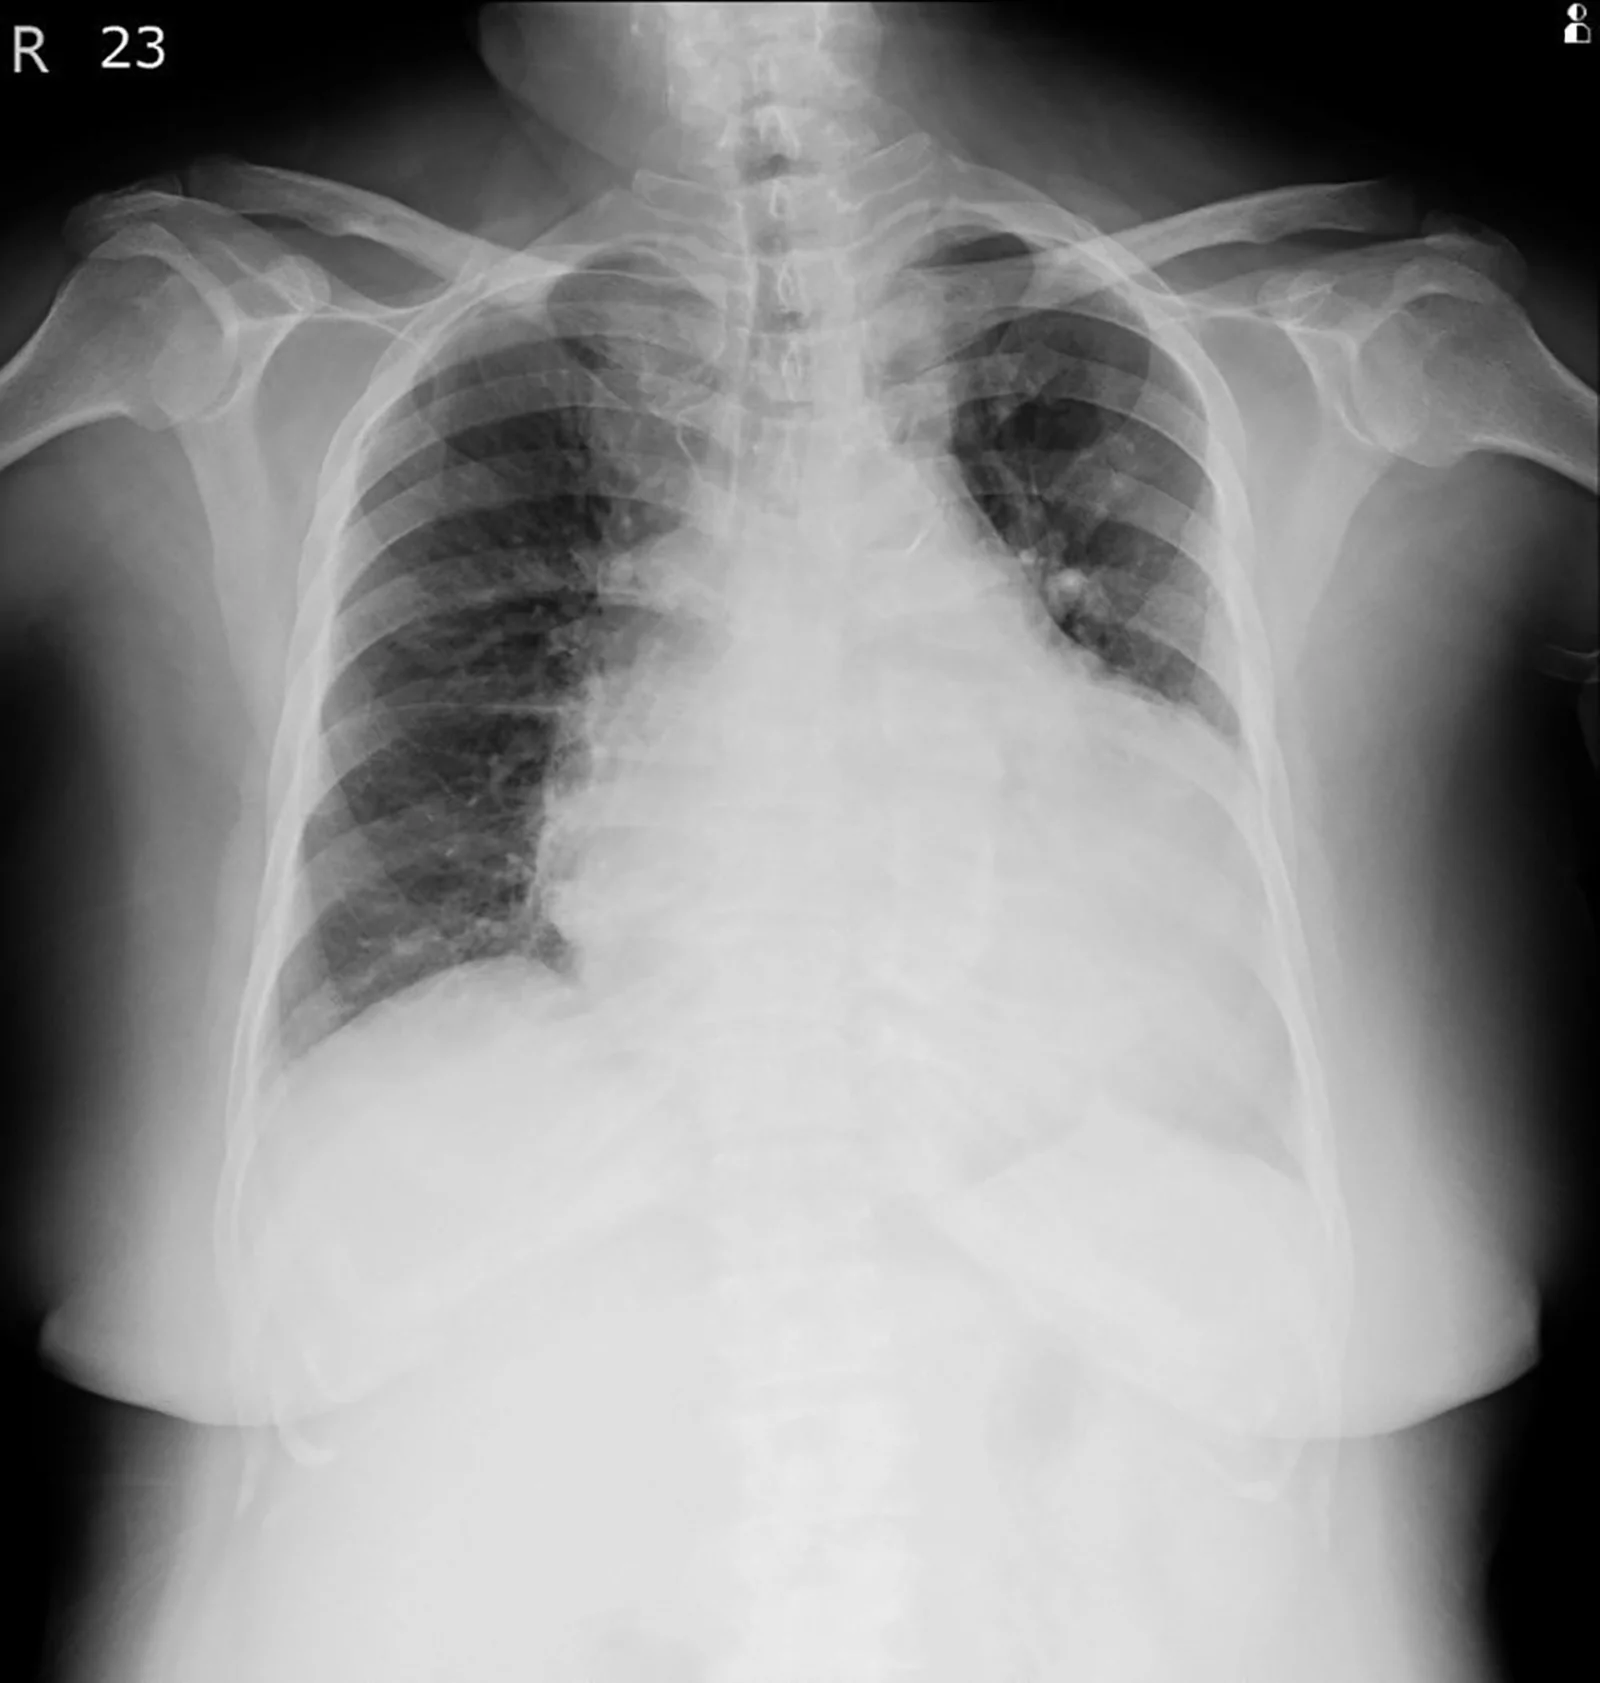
Chest radiograph demonstrating the characteristic “water-bottle” silhouette of the heart.
